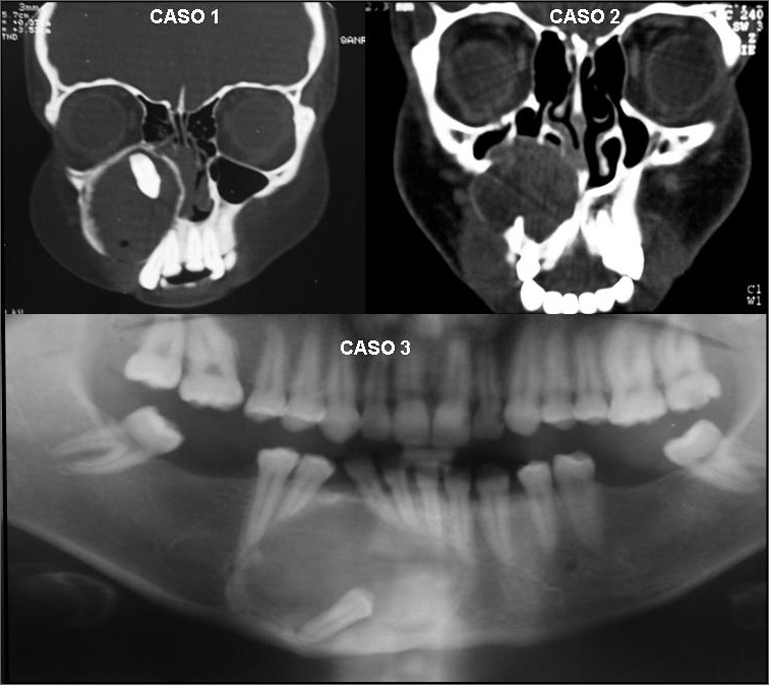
Radiographic aspects of cases 1, 2 and 3.
